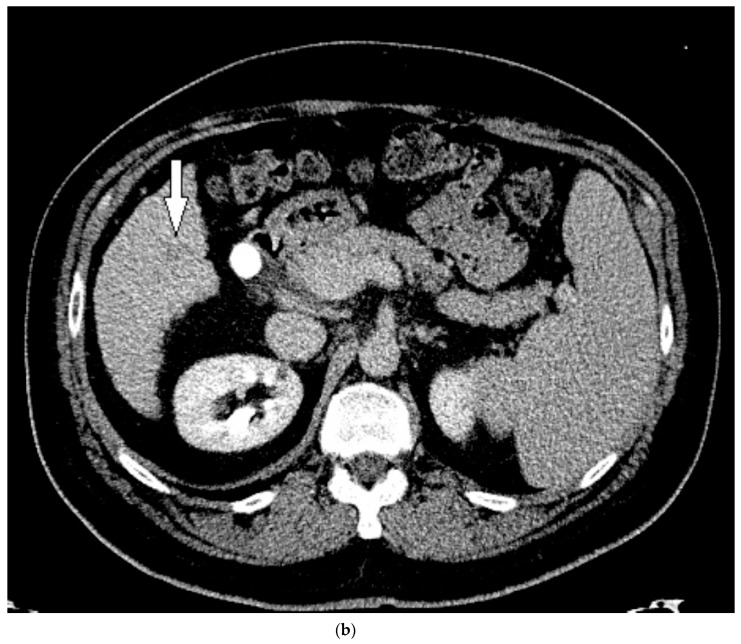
Patient 2 CT images using liver windows to highlight the lesion seen on CEUS (white arrow): (a) late arterial phase axial contrast-enhanced CT; (b) 3 min delayed phase.
